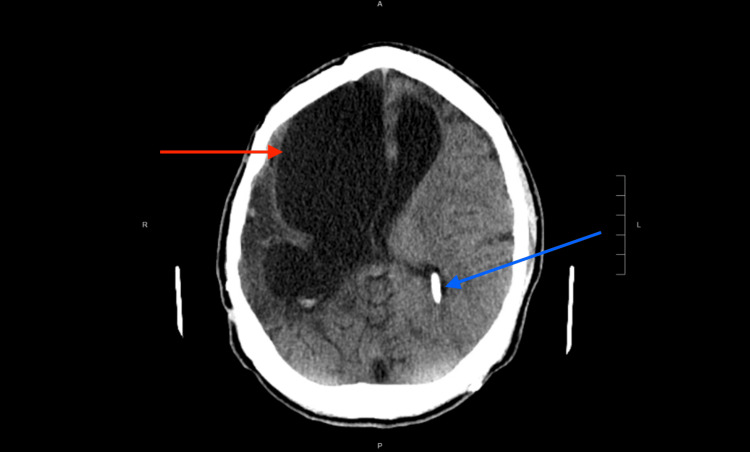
Recent non-contrast head CT Red arrow: cystic encephalomalacia in the right cerebral hemisphere. Blue arrow: left posterior approach ventriculostomy.
